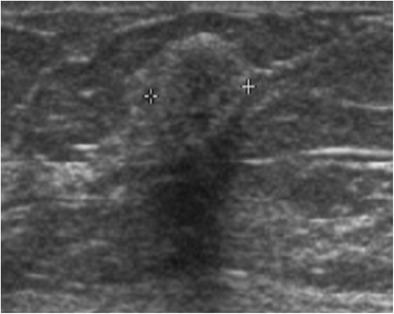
Myofibroblastoma. Ultrasound scan showing a slightly heterogeneous, hyperechoic and attenuating rounded mass
